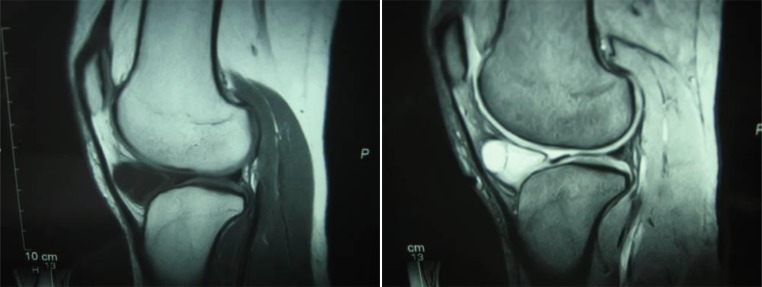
MRI showed a multilobular cyst in the infrapatellar fat pad with T1 low intensity and T2 STIR high intensity. The cyst was not attached to either menisci.
